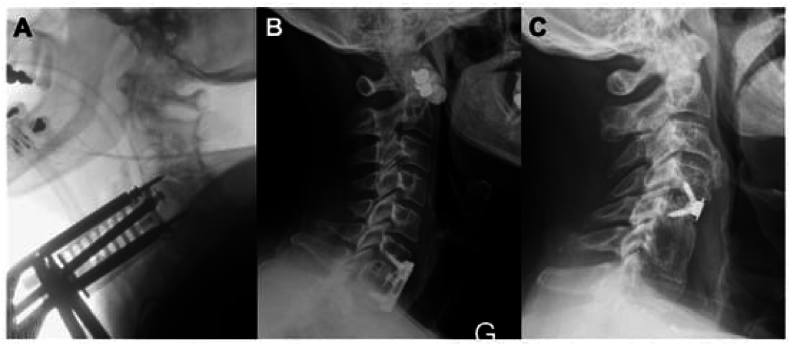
Anterior cervical cage placement and fixation. (A) Intraoperative lateral X-ray to assess ACDF cage placement, with Casper pins shown. (B) Post-operative lateral X-ray showing a single level ACDF at C6–C7 secured by a plate (Venture, Medtronic). (C) Post-operative lateral X-ray showing a single level ACDF at C4-5 (Zero-P® cage, DePuy Synthes). Adapted from Sommaruga et al.^5^ 2021 under the under the Creative Commons Attribution-Non-Commercial License 4.0 (CCBY-NC).
